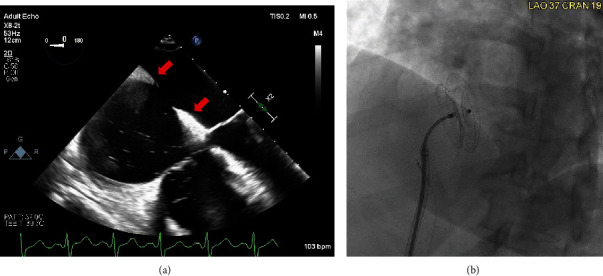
(a) TEE mid-esophageal four-chamber view confirming ASD with an adequate rim for percutaneous device closure (arrow). (b) Percutaneous deployment of a 26 mm Amplatzer Septal Occlusive device. ASD: atrial septal defect; TEE: transesophageal echocardiogram.
